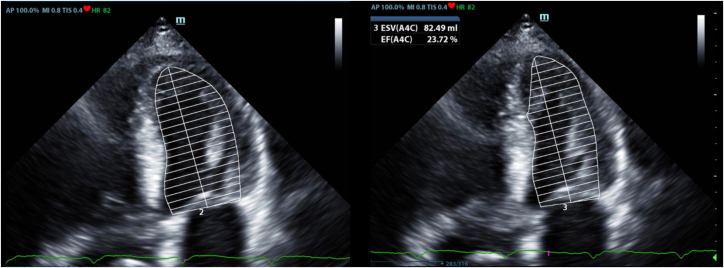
Echocardiogram (Diastolic Image on the Left, and Systolic Image on the Right) Showing a Left Ventricular Ejection Fraction of Approximately 25%
